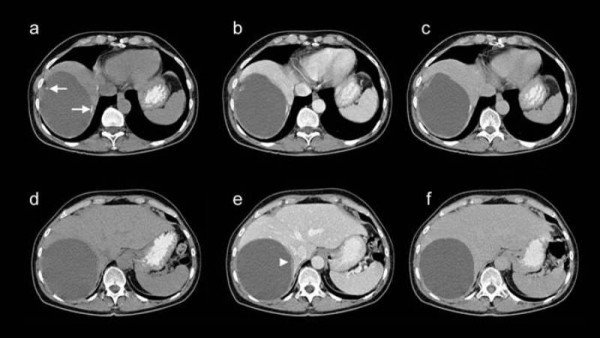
Transverse computed tomography, non-enhanced (a, d), arterio-venous-enhanced (b, e) and late-enhanced (c, f), images. Upper row of images demonstrating the calcifications in the cyst's wall (arrows), lower row depicting the enhancement of the cyst's wall (arrowhead).
